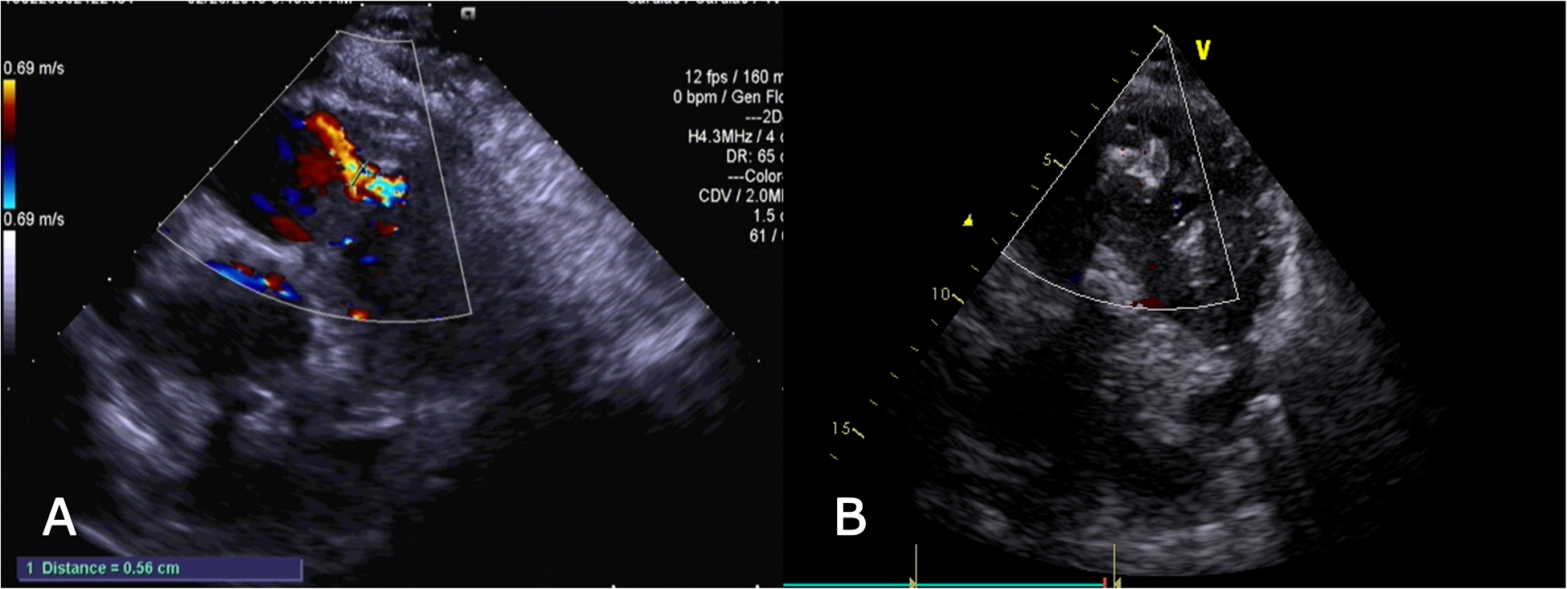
a Preoperative echocardiography shows muscular ventricular septal defect. b Postoperative echocardiography shows that the occluder is in a good position with no residual shunt
